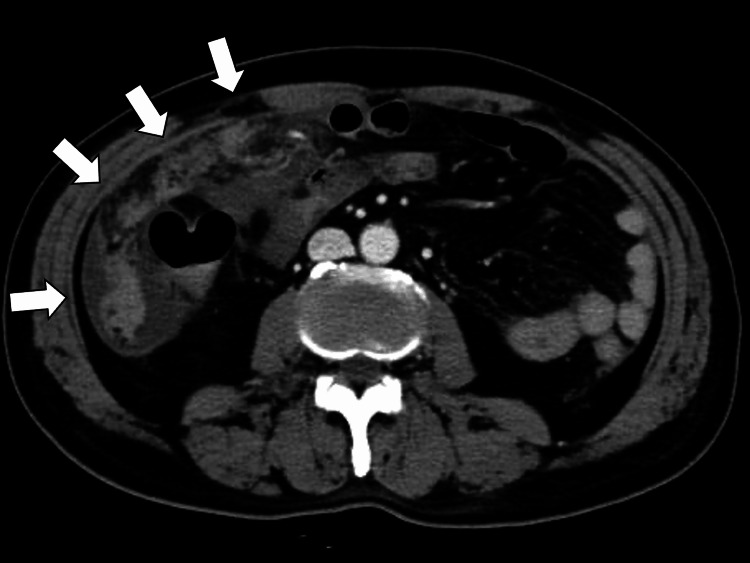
Abdominal computed tomography revealing newly developed ascites and peritoneal thickening (white arrows)
